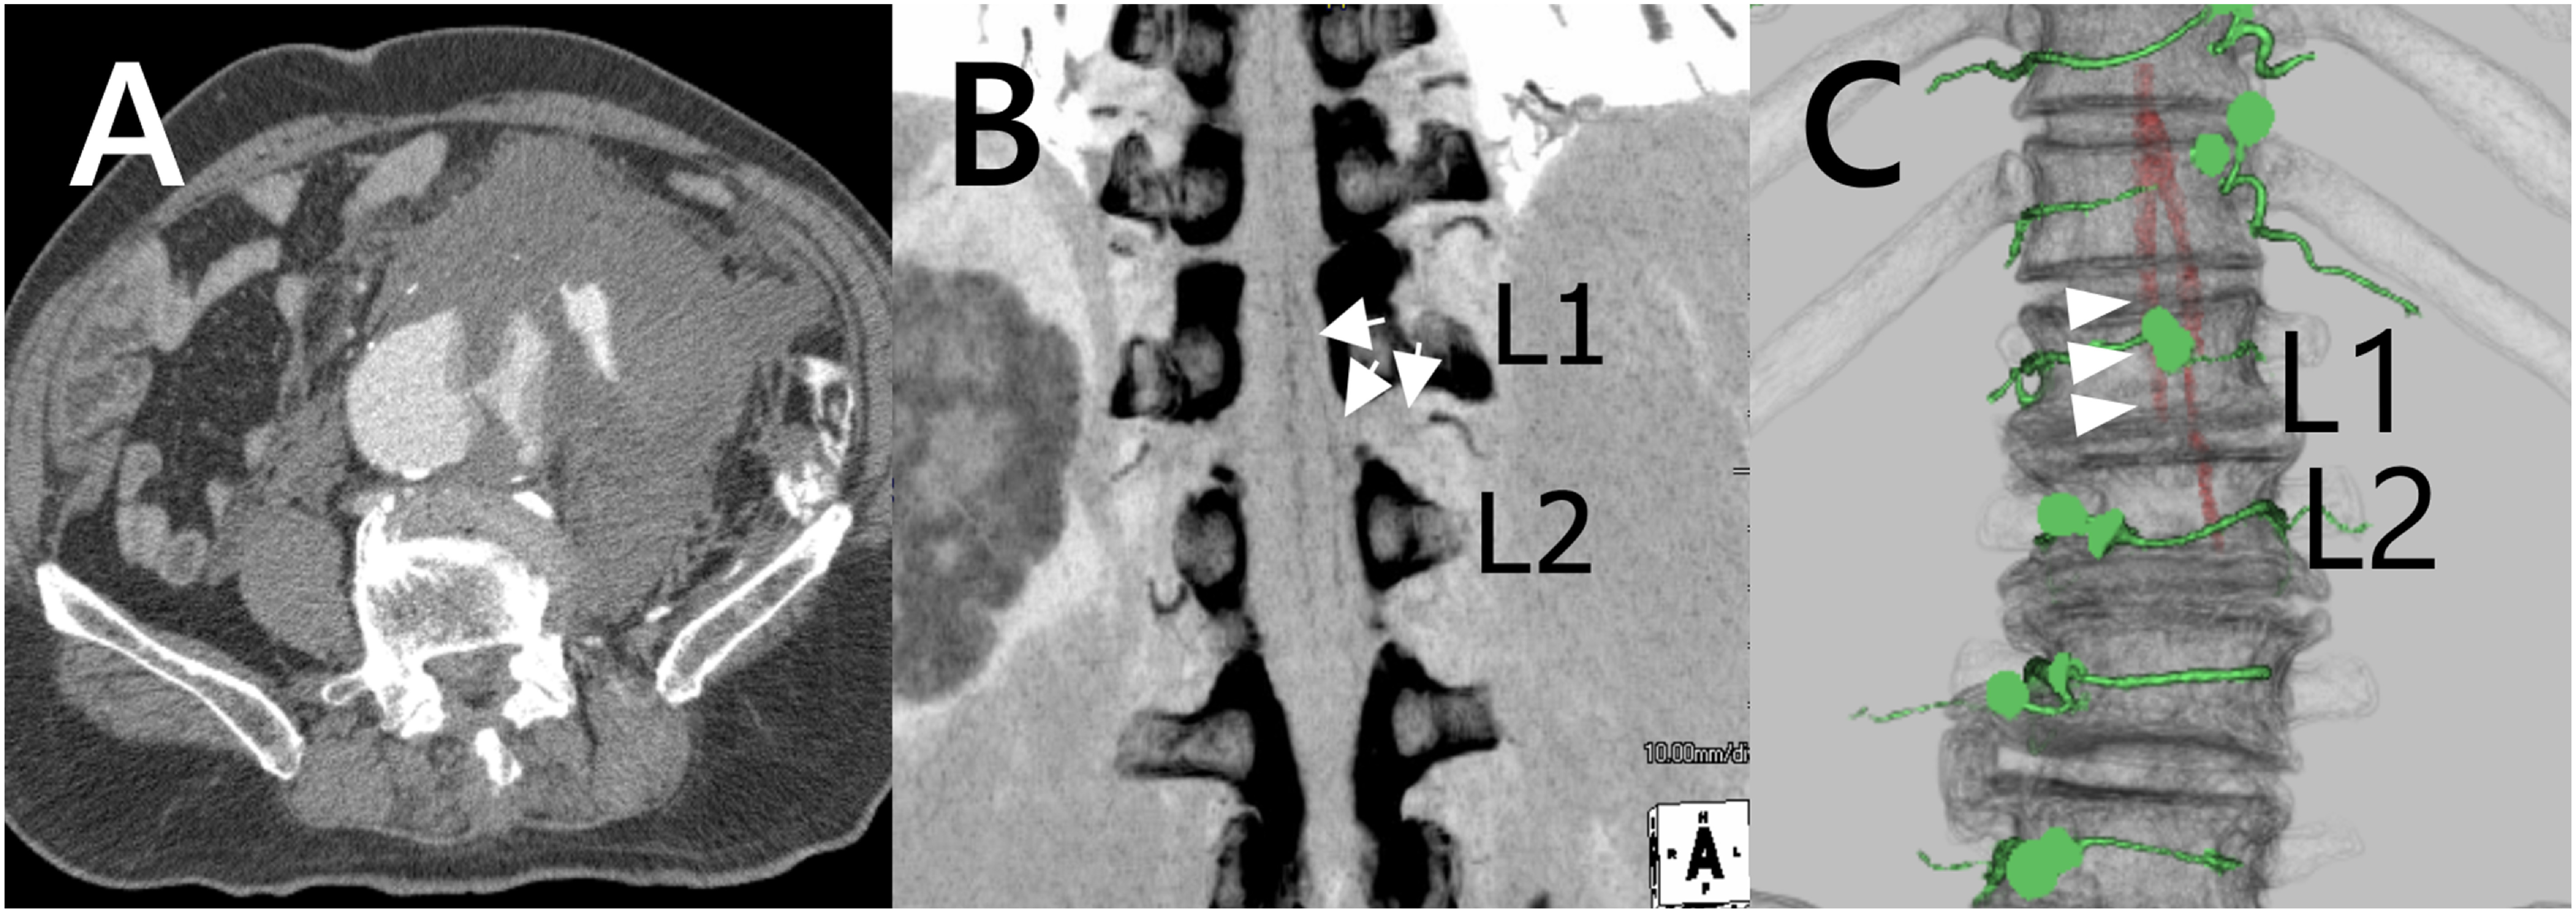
Fig. 1 (A) Preoperative computed tomography reveals a 51 mm serial left common iliac artery aneurysm from the abdominal aortic aneurysm and a retroperitoneal hematoma. (B) Preoperative contrast-enhanced computed tomography showing the Adamkiewicz artery originating at the L1–L2 level (white arrows). (C) Three-dimensional computed tomographic reconstruction: The Adamkiewicz artery is indicated by white arrow heads.
